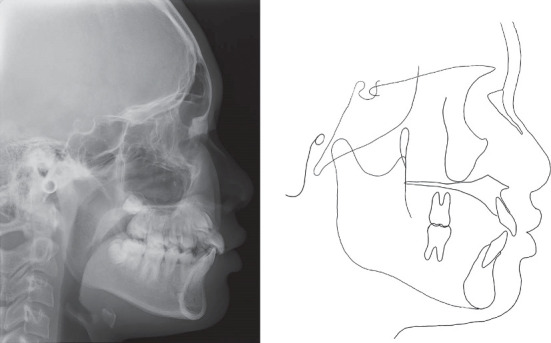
Pretreatment lateral cephalometric radiograph and cephalometric tracing.
